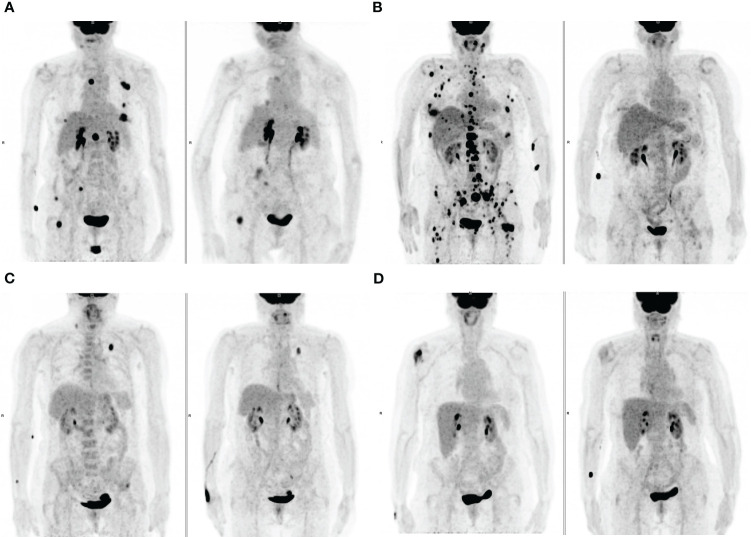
Maximum-intensity projection (MIP) FDG PET images at baseline (left) and at first PET evaluation during treatment (right). (A) An 83-years old patient with lymph node and bone metastases and a high TMTV1 (50.9 cm3) showing PMR at PET2 with a Deauville score >3 (5). (B) a 72-years old patient with lymph node, bone and liver metastases and a high TMTV1 (212.4 cm3) showing PMR at PET2 with a Deauville score ≤ 3 (3). (C) A 75-years old patient with metastases in the left lung, left iliac bone and in a right supraclavicular lymph node with low TMTV1 (8.3 cm3) showing PMR at PET2 with a Deauville score >3 (4). (D) A 73-years old patient with a single metastasis in the right humeral head with low TMTV1 (7.7 cm3) showing PMR at PET2 with a Deauville score ≤ 3 (3).
